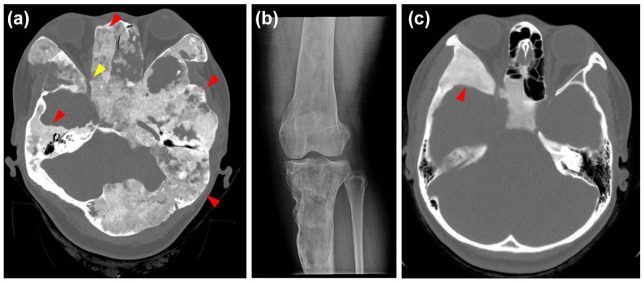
Radiologic imaging of fibrous dysplastic bone. (a) Craniofacial CT scan of a 33-year-old female with FD/MAS, showing extensive fibrous dysplastic bone in the skull (representative lesions, red arrows). Narrowing of the optic canal can be seen in this image (yellow arrow). In addition, this craniofacial lesion shows cystic changes that can be seen in some FD lesions. (b) FD of the right tibia as seen on plain film X-ray, of the same patient at age 29. (c) FD of the craniofacial region in a 16-year-old female, showing the classical ground glass-like lesion. CT, computed tomography; FD, fibrous dysplasia; MAS, McCune-Albright syndrome.
